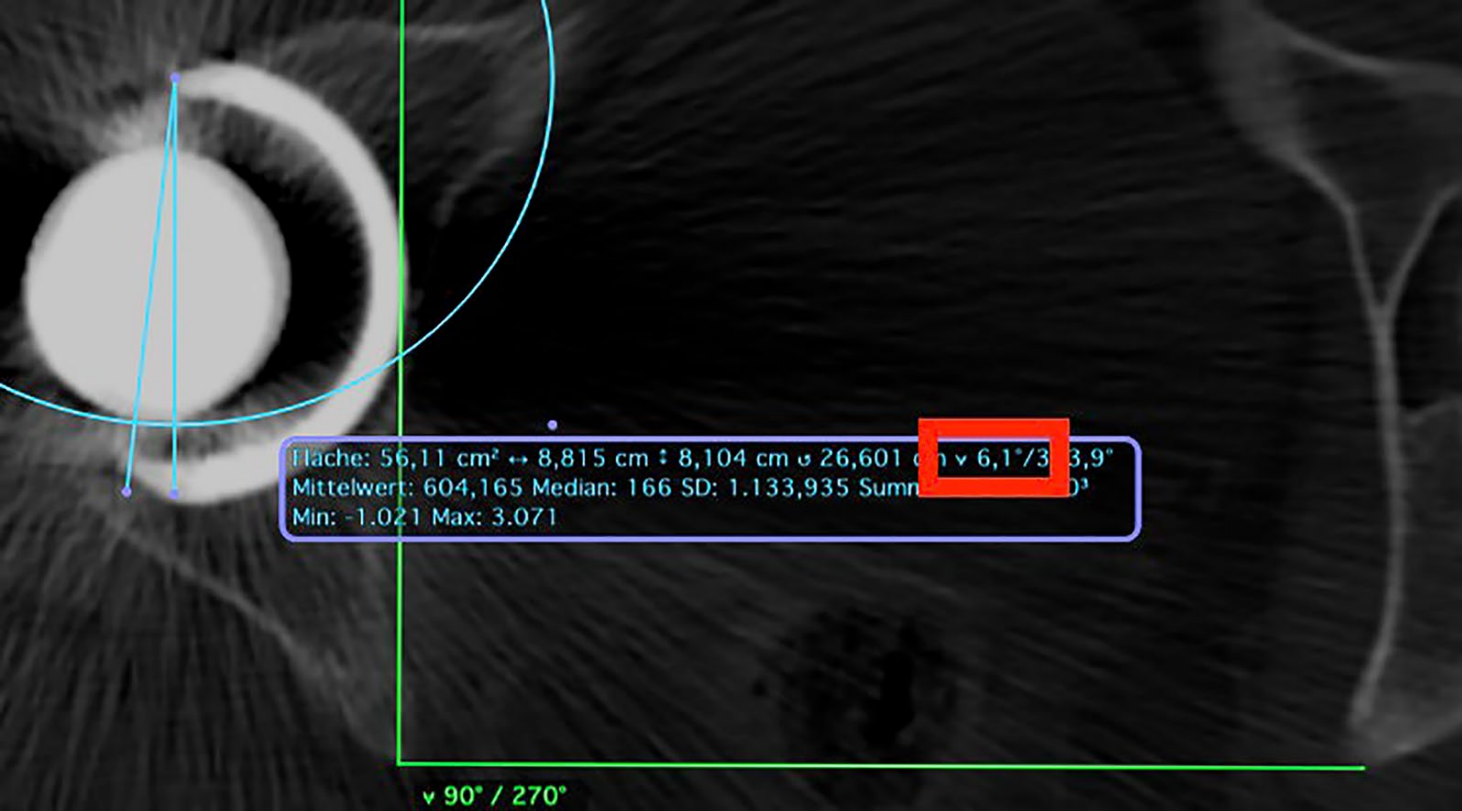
Rotational CT scans of the lower extremities were assessed using the software OsirixLite (Pixmeo, Bernex, Switzerland). In the provided example, the cup’s anteversion is measured
